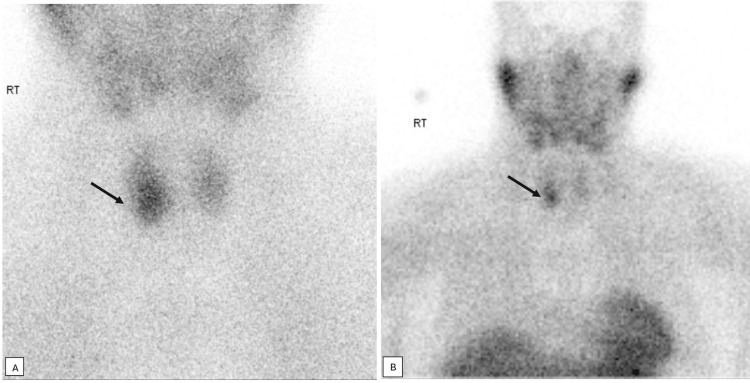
Parathyroid SPECT. 11 mm soft tissue nodule (black arrows) abutting the right cervical paravertebral muscles posteriorly and the trachea medially at the level of right mid/lower pole thyroid, with increased tracer uptake. SPECT: single-photon emission computed tomography.
